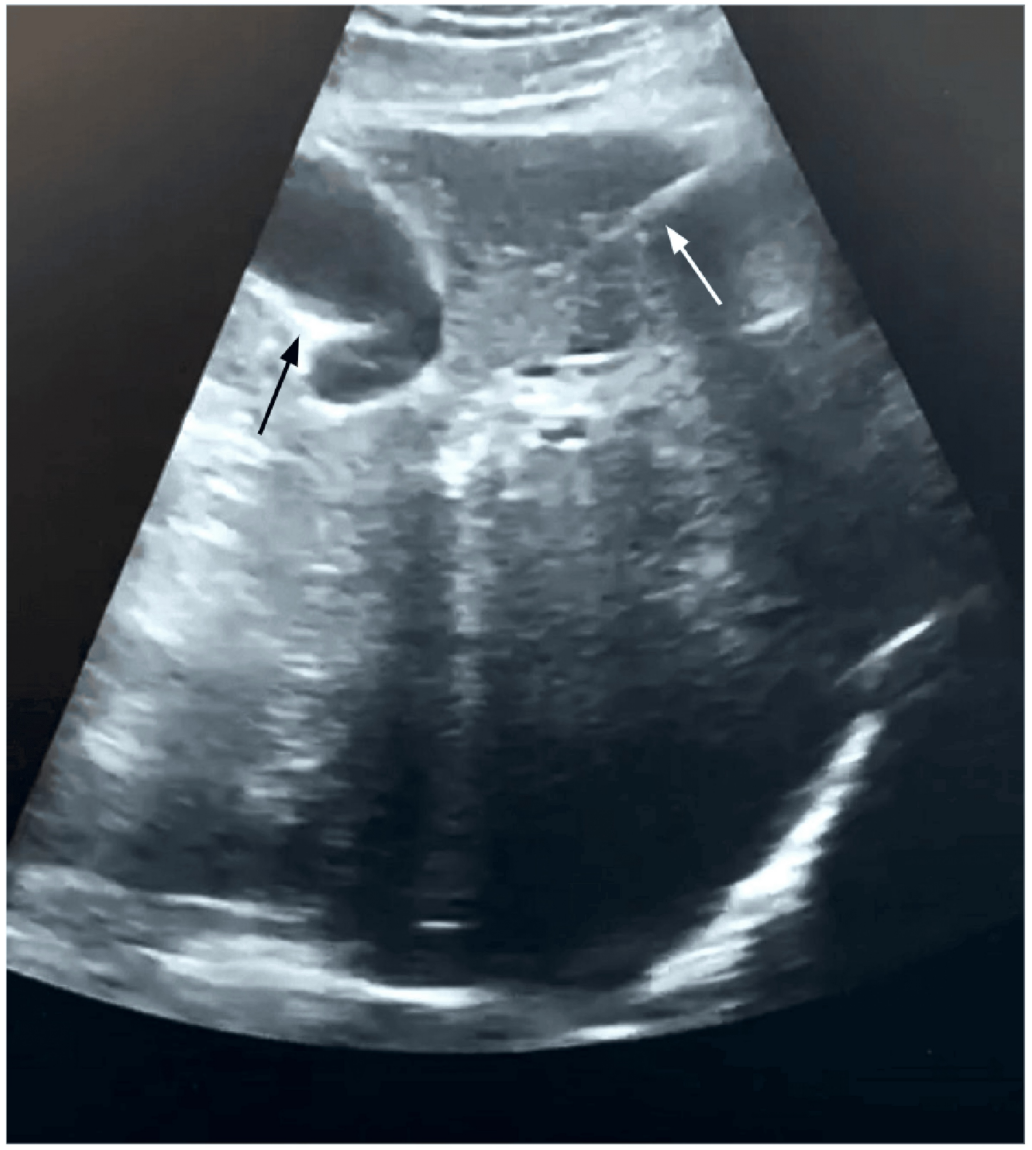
Intraoperative ultrasound showing the foreign body (white arrow) and the gall bladder (black arrow).
